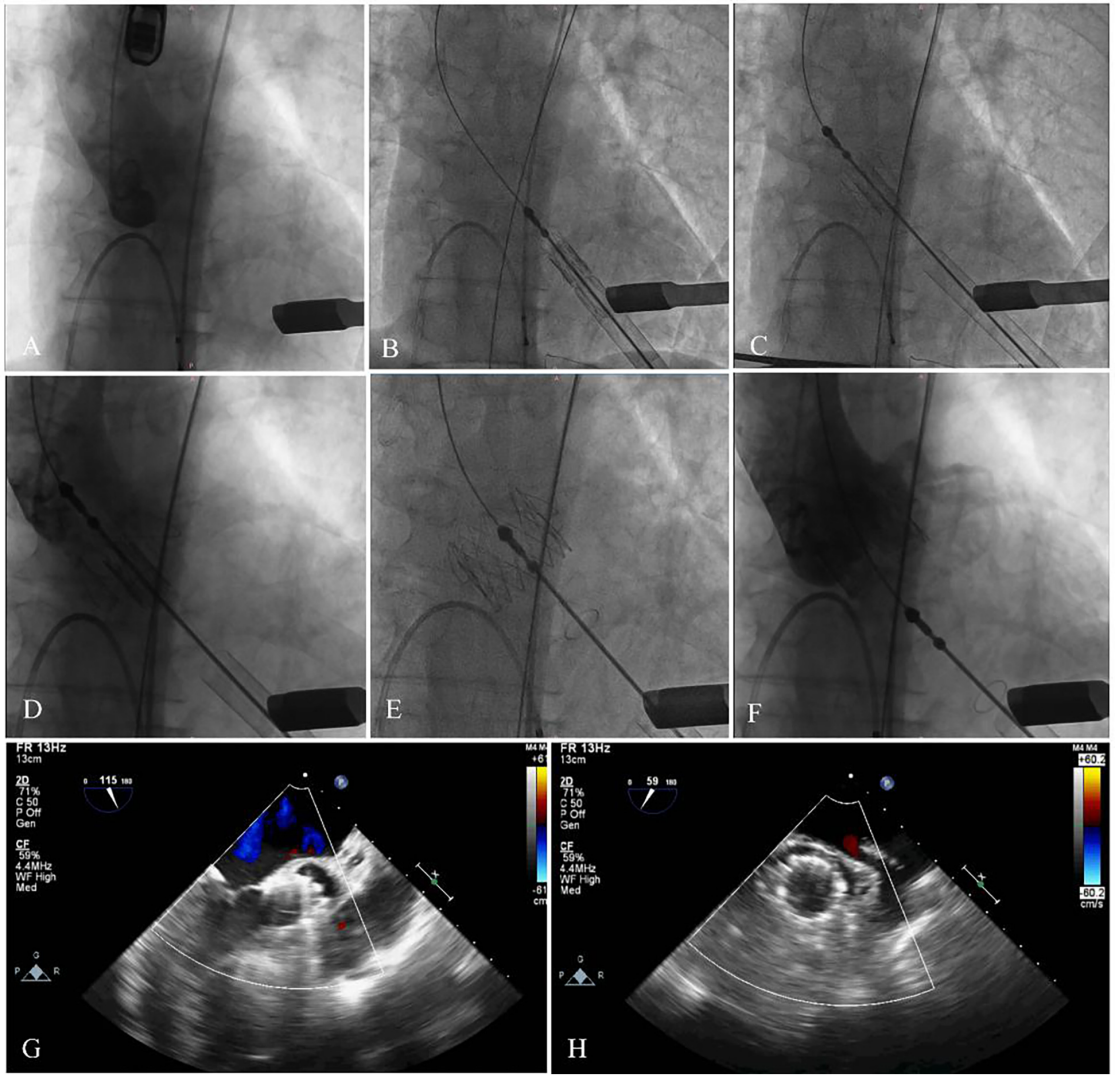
The main steps of the procedure. (A) Fluoroscopy showed the position of aortic root. (B) The delivery system was advanced via the transapical approach. (C,D) The delivery system was advanced. (E,F) The stent was fully released, and fluoroscopy revealed that the bioprosthesis was in a stable position and functioning well with no paravalvular leak and coronary artery occlusion. (G,H) Echocardiography showed no regurgitation, and excellent leaflet mobility.
